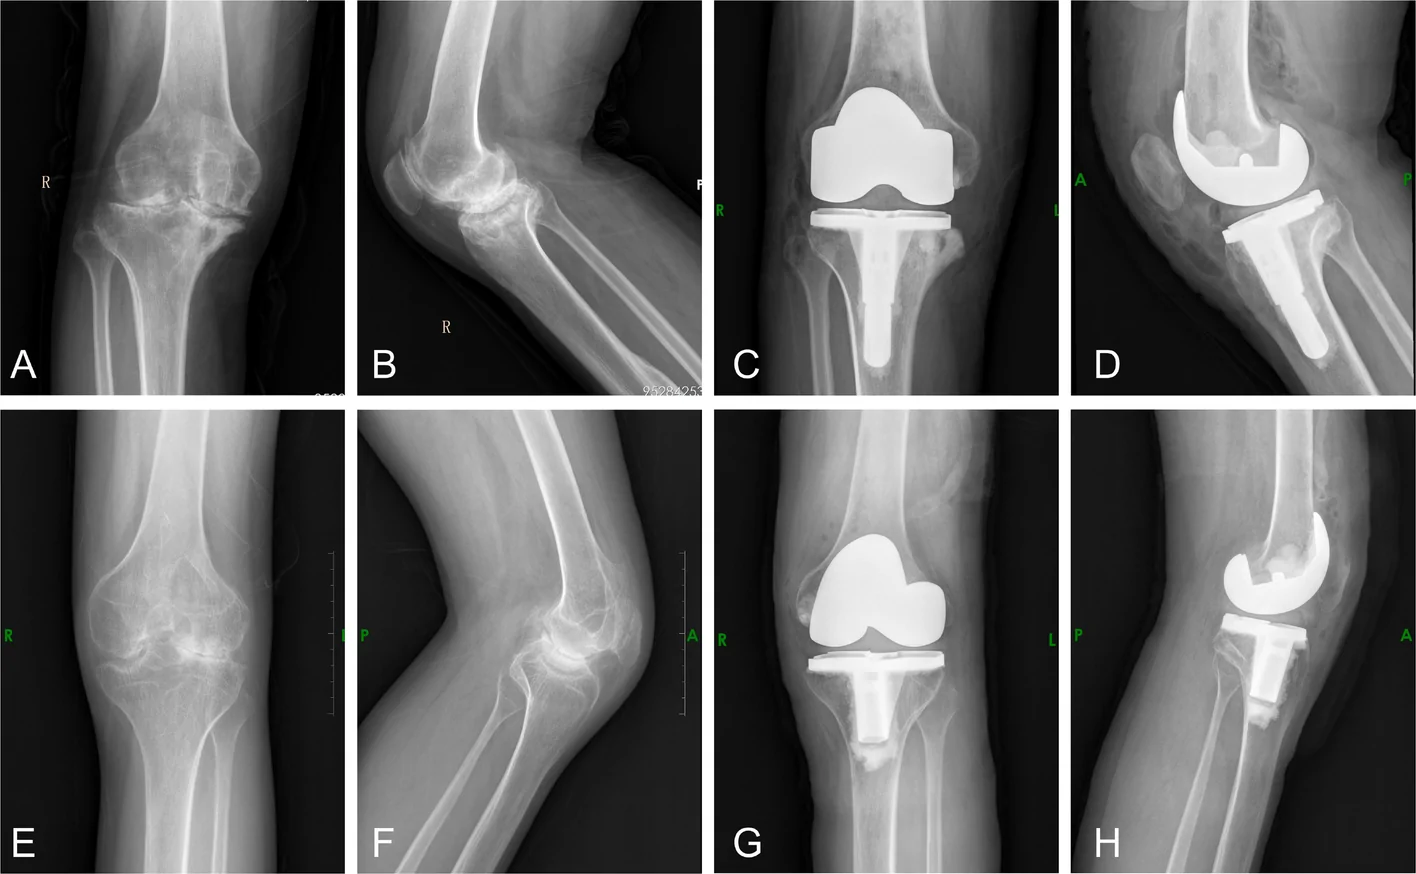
A–D Preoperative and postoperative anteroposterior and lateral X-rays of the knee joint in a patient with hemophilia A. E–H Preoperative and postoperative anteroposterior and lateral X-rays of the knee joint in a patient with hemophilia B
